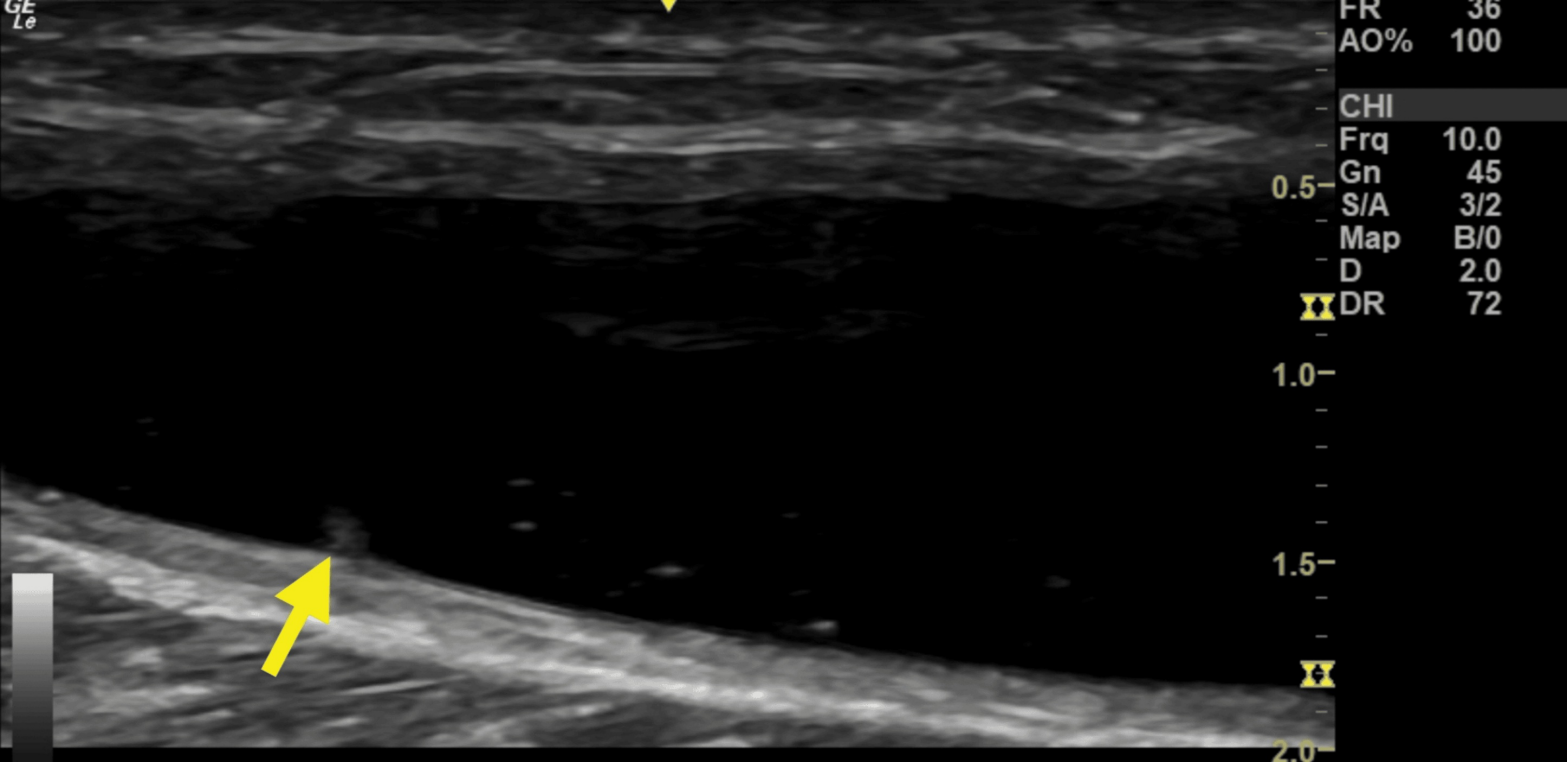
Sonographic image demonstrating sagittal, long axis view of anechoic, compressible fluid collection located 4-8 cm distal to the level of the popliteal fossa.
